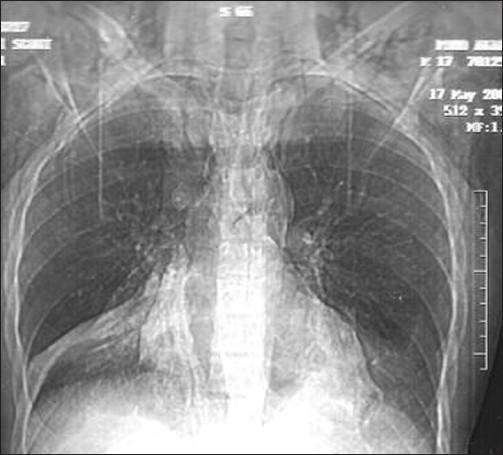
Chest x-ray showing extensive subcutaneous emphysema and pneumomediastinum
